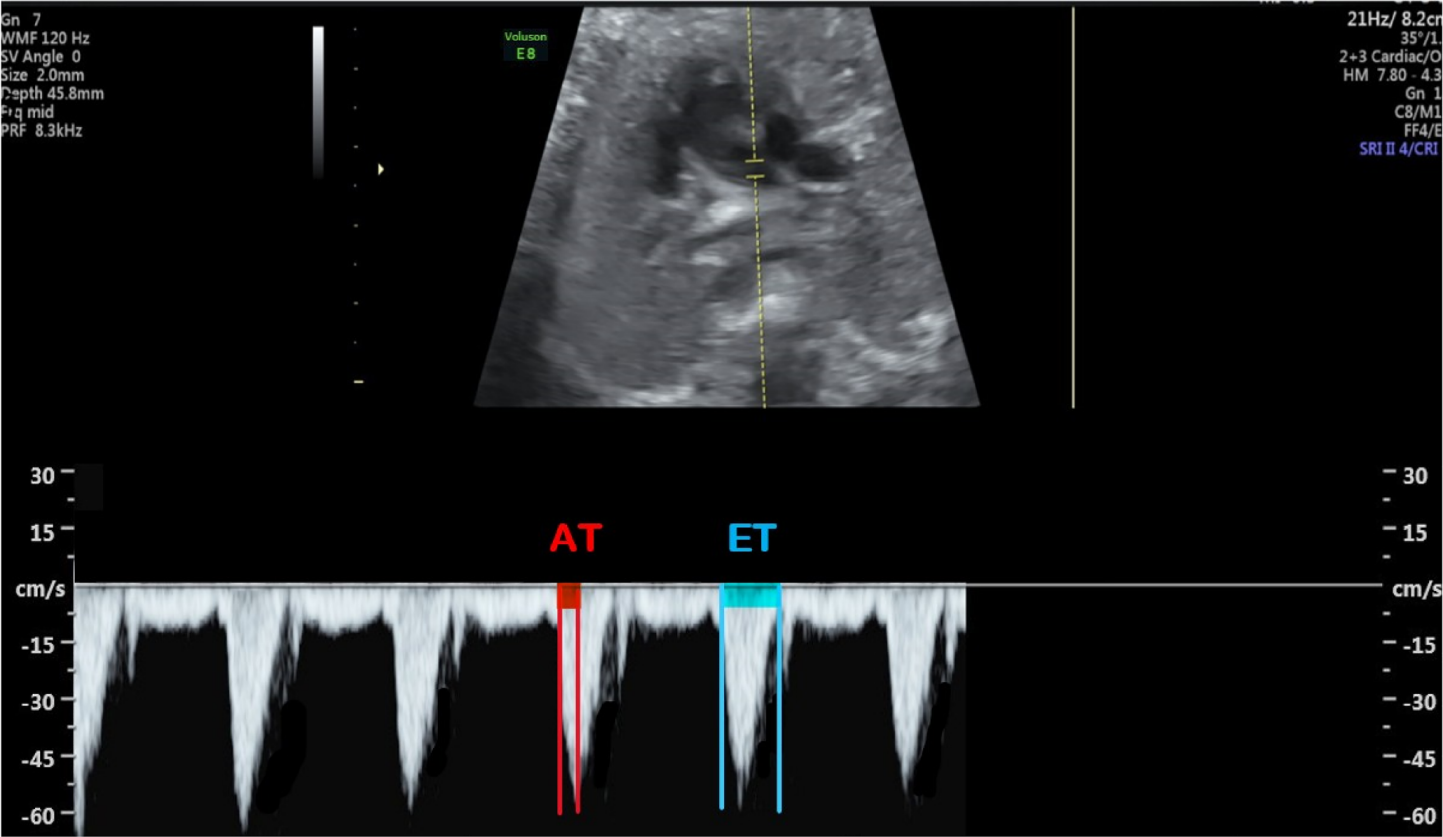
Right pulmonary artery Doppler parameter. AT stands for acceleration time, which is the time between the start of systole and its peak, and ET stands for ejection time, which is the time between the start of systole and its end.
